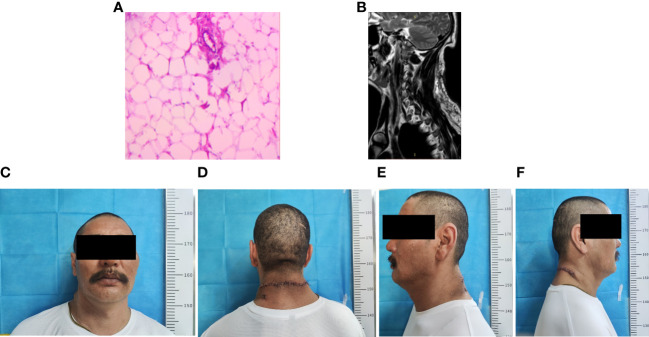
Postoperative clinical and imaging findings. (A) Postoperative pathological findings (B) Postoperative neck MRI showing complete excision of the subcutaneous neck mass. (C–F) Postoperative clinical images of the patient.
